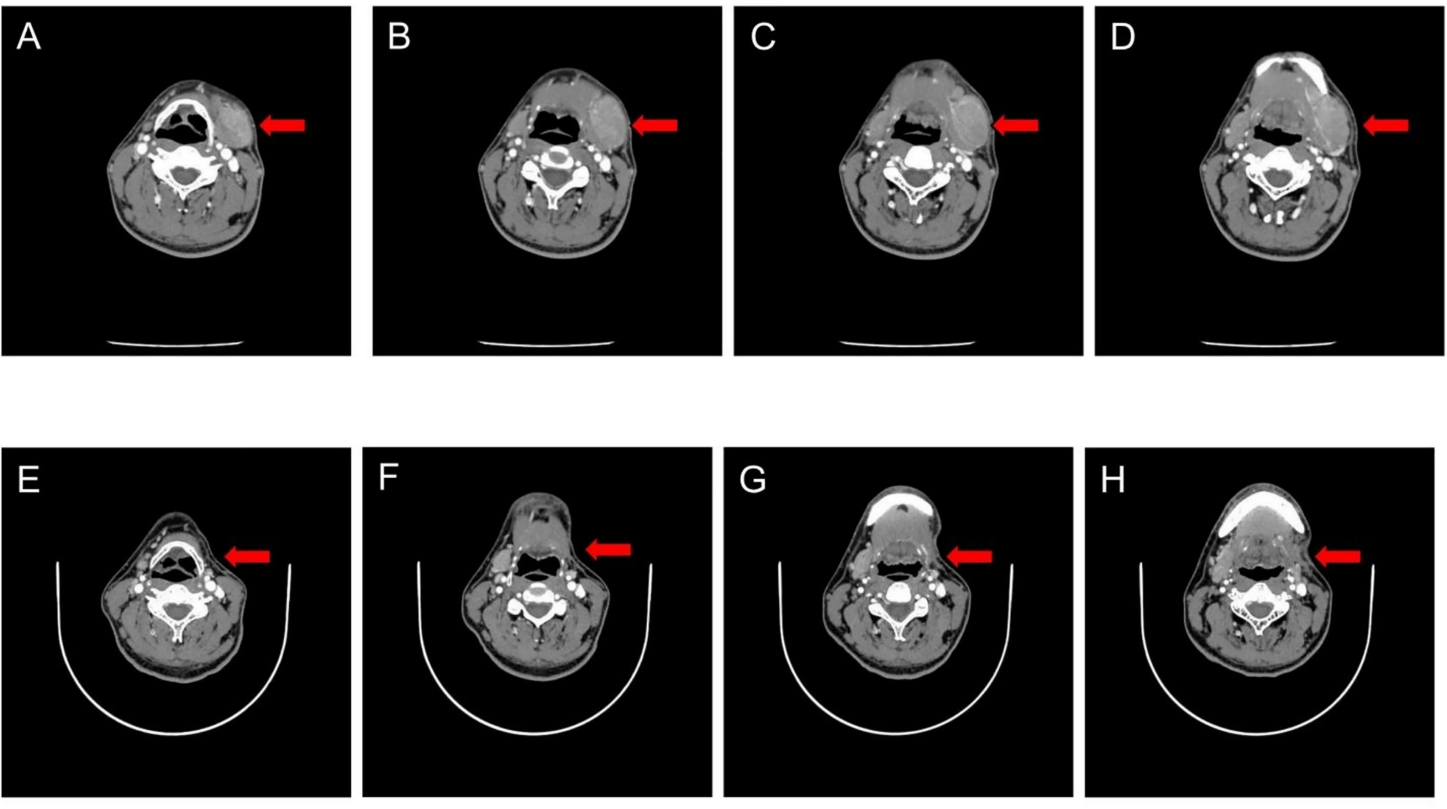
Representative contrast-enhanced CT images of a patient with AIDS-related non-Hodgkin lymphoma (AR-NHL) before and after four cycles of R-CHOP chemotherapy. Panels (A–D) represent contrast-enhanced CT images of the patient’s neck obtained at initial diagnosis; panels (E–H) show follow-up contrast-enhanced CT images of the neck acquired after 4 weeks of treatment. The left submandibular lymphoma of the patient showed a marked regression after treatment.
